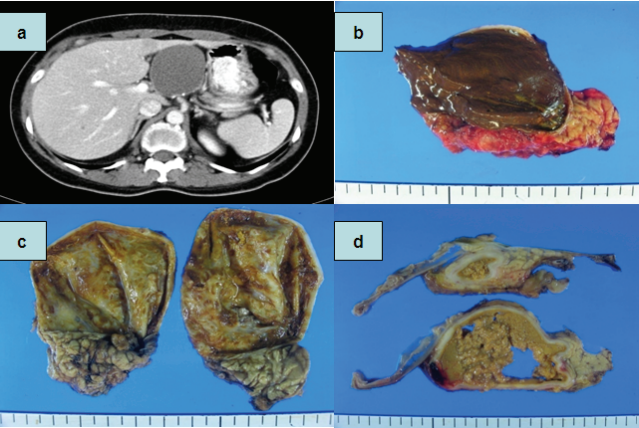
Solid pseudopapillary tumor with cystic degeneration. (a) CT shows cystic mass in pancreas head portion. (b-d) Gross appearance with cyst contains chocolate like materials suggestive with necrotic debrids.
